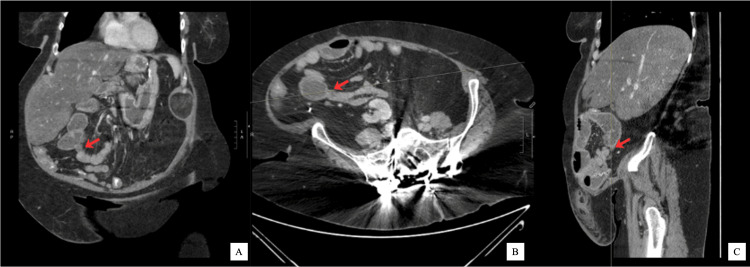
Case 2: Coronal (A), axial (B), and sagittal (C) views of a CT abdomen/pelvis demonstrating dilated loops of small bowel with a transition point at the surgical anastomosis, as well as postoperative changes and fat stranding in the abdominal wall. This CT abdomen/pelvis shows dilated fluid-filled small bowel extending from the gastrojejunostomy site with a transition point in the right lower quadrant (red arrows) at the surgical anastomosis. CT: computed tomography
